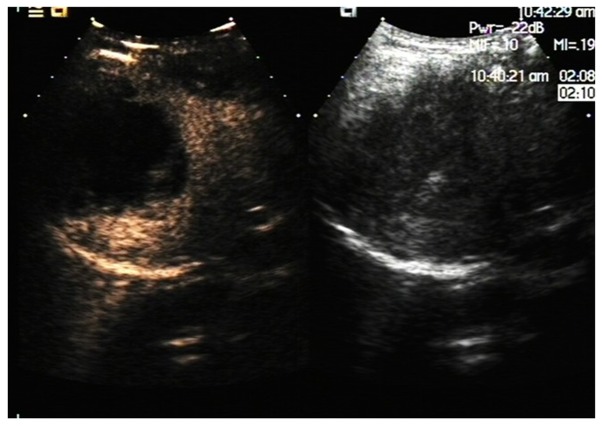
A contrast-enhanced ultrasound showing that the liver capsule is smooth, the size is normal and the internal echo is uniform. There is a 90×67 mm echo in the right liver lobe, with a clear boundary and shape. The contrast-enhanced ultrasound shows a clear boundary, circular enhancement in the arterial phase, no enhancement in the center, tantamount enhancement in the portal vein and a delayed phase.
